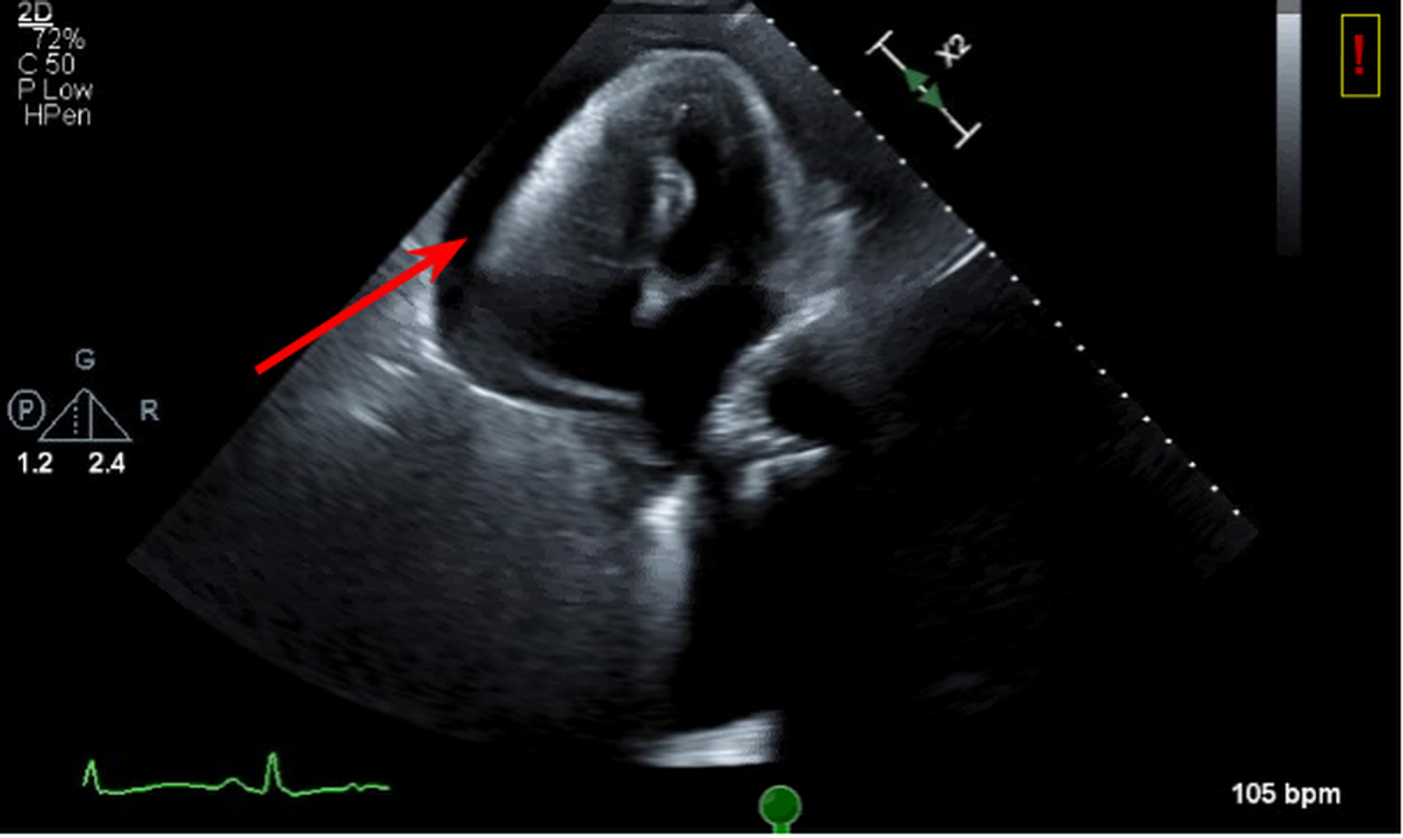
2D echocardiogram on apical view showing fluid around the right atrium and ventricle with a completely collapsed right ventricle (arrow).
